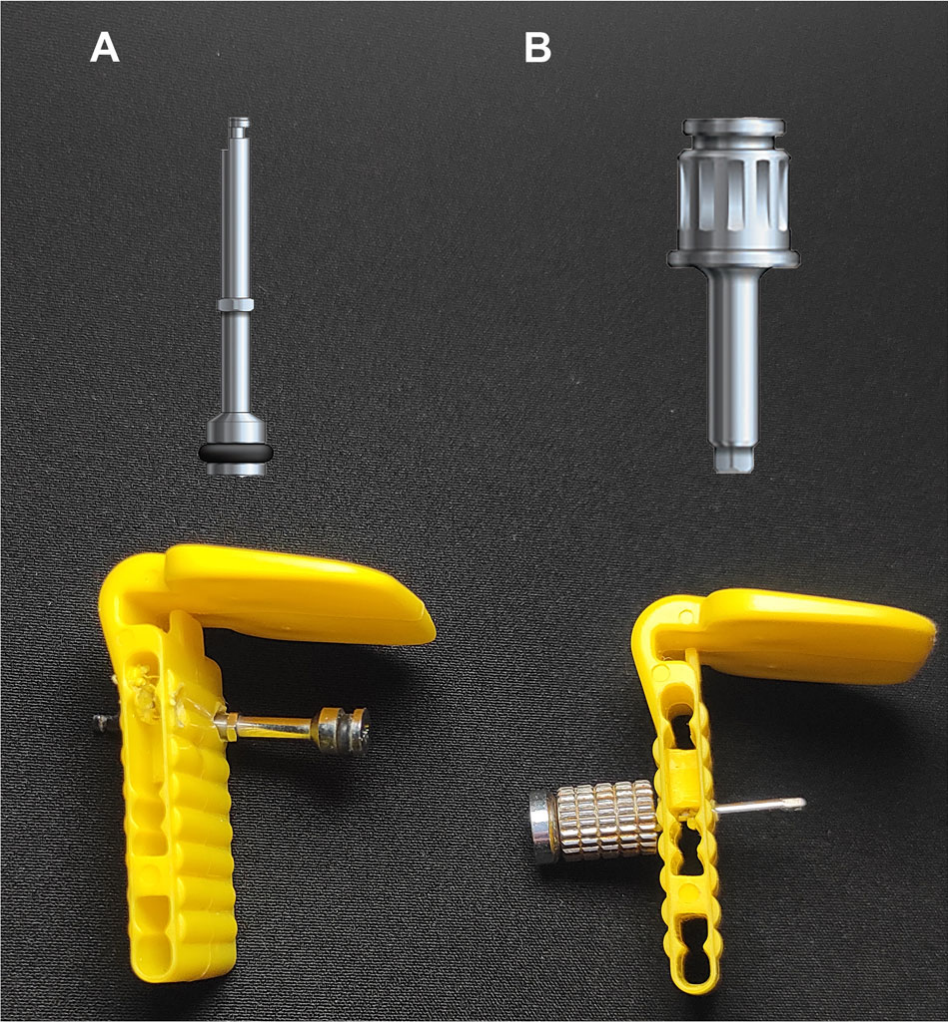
Modified X-ray sensor holder for parallel periapical images of mandibular implants. A for mini-implants, B for standard implants.
